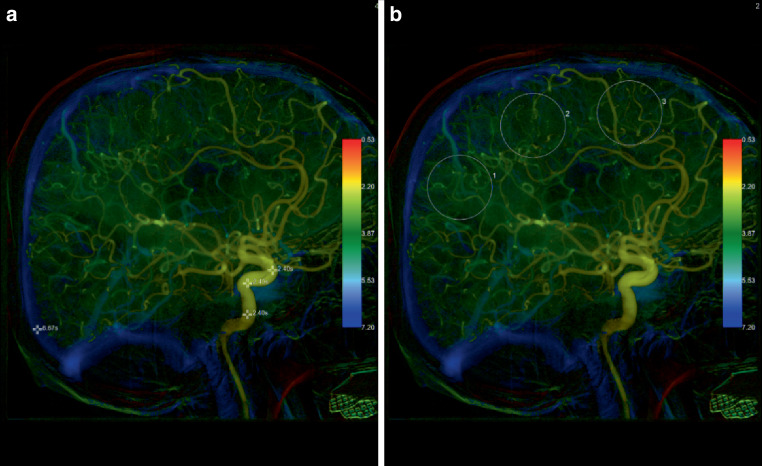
A color-coded image from a lateral 2D DSA series of a 75-year-old patient. Analogous to Fig. 1 POIs and ROIs were placed. This patient was discharged from hospital with an NIHSS score of 2 with only a minor infarction on subsequent CT imaging. The microvascular transit time (*mTT*) was calculated to be 2.40 s
